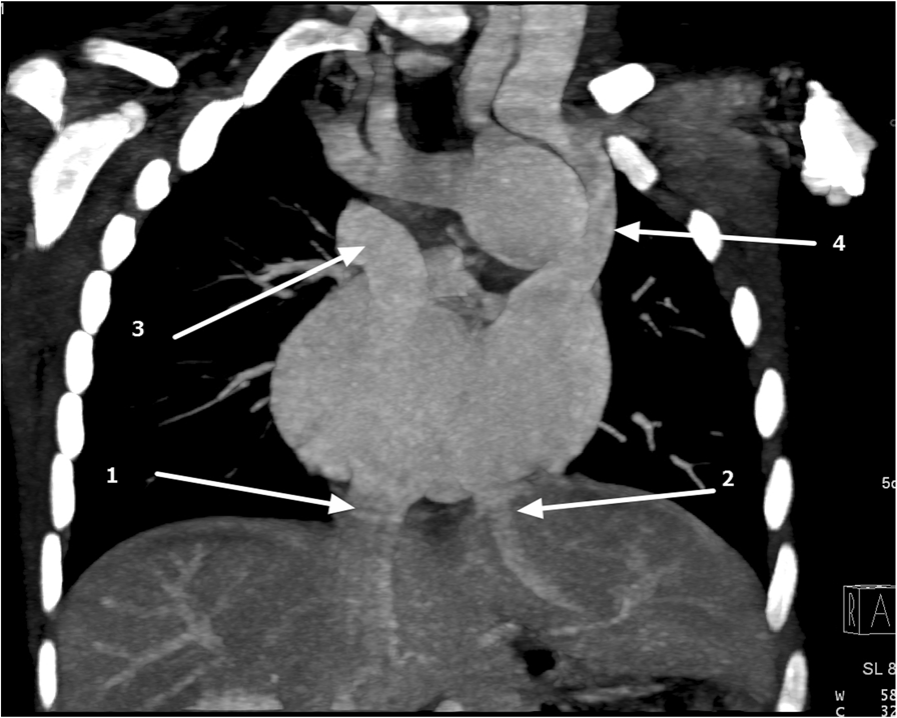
Coronal maximum intensity projection computed tomography showing asplenia, inferior vena cava (1) draining into right-sided atrium and left hepatic vein (2) draining into left-sided atrium and double-sided superior vena cava draining into separate atria (3 and 4)
